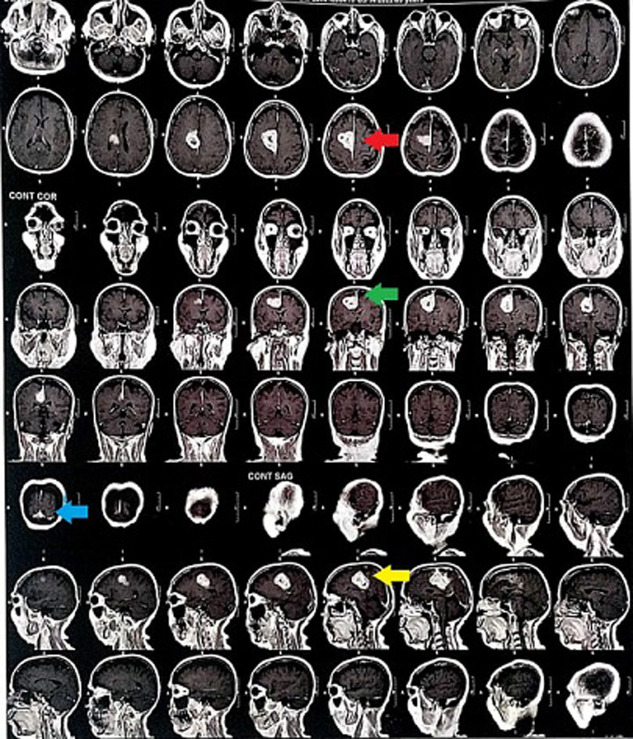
MRI findings of grade II meningioma; red and green arrows show extra-axial lesions measuring approximately 4.1*3.9cm; the blue arrow shows the mass effect; the yellow arrow shows neoplastic etiology
